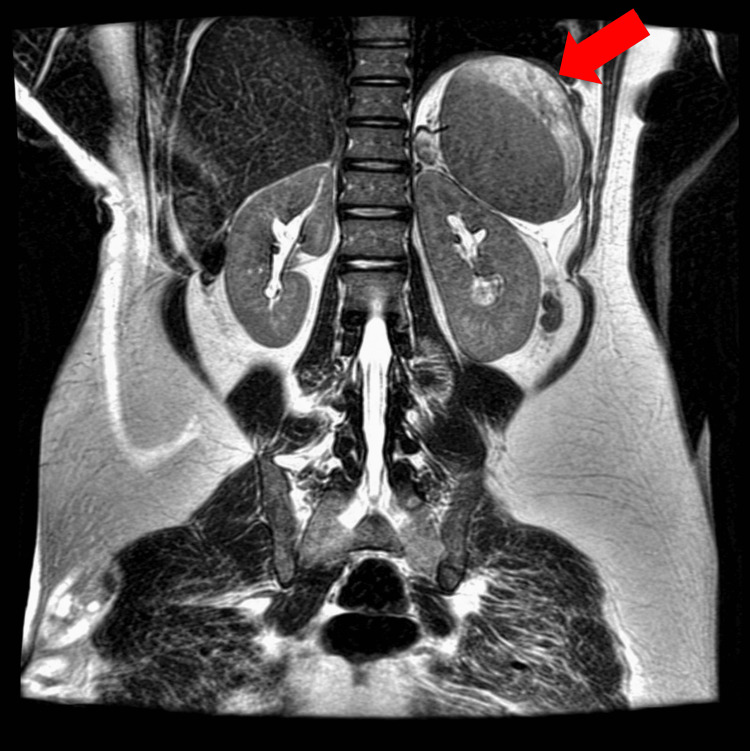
MRI of the abdomen and pelvis showing splenic hemorrhage The spleen measures 10.2 cm in length. The red arrow points to the perisplenic and subdiaphragmatic fluid collection, which demonstrates intermediate signal intensity on T2-weighted images.
